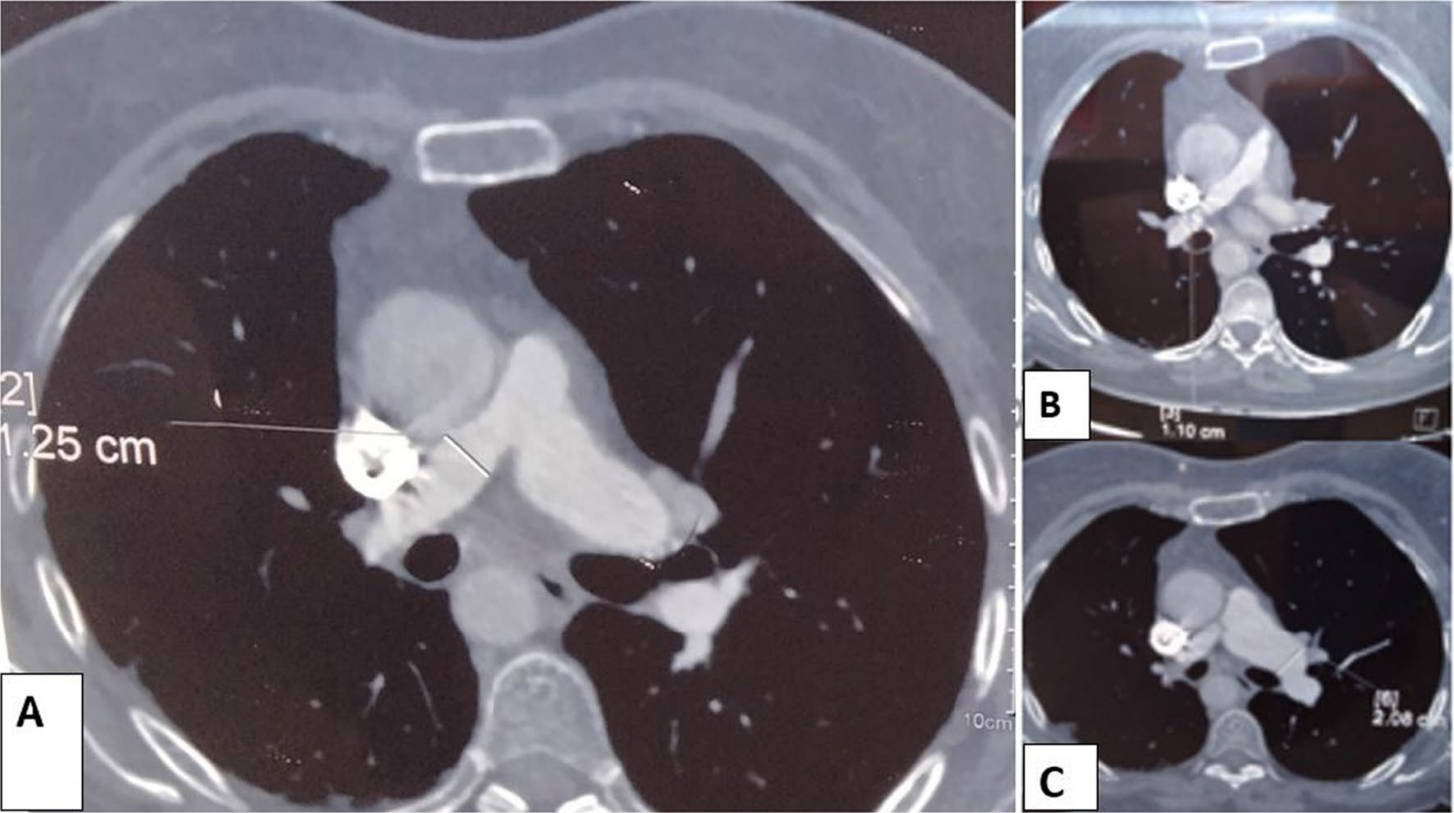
The dimensions of the pulmonary branches proximally (A) and peripherally (B and C) on the CT angiography of the chest
